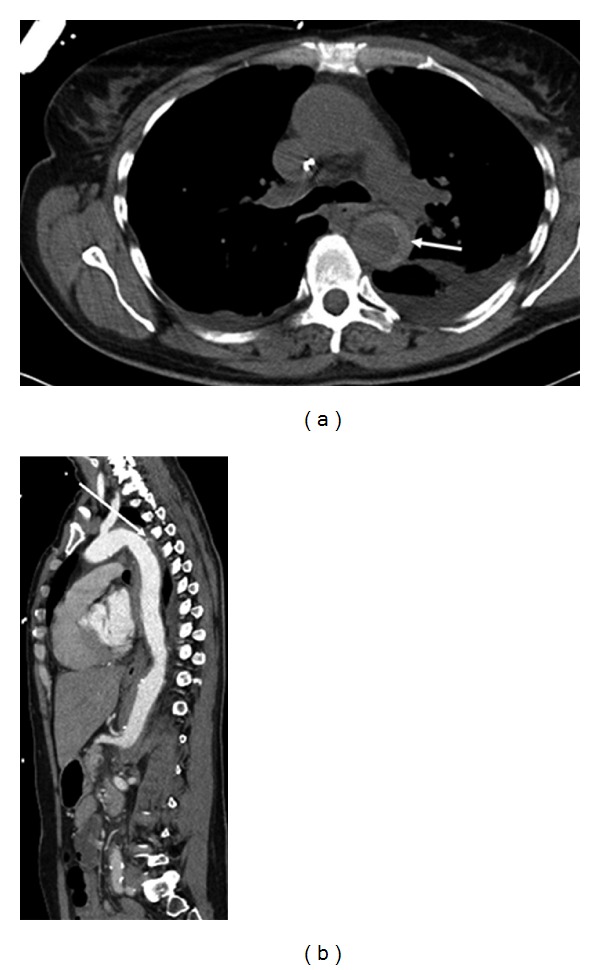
(a) Nonenhanced CT depicting intramural hematoma (arrow). (b) Sagittal reconstruction of CT-angiography (CTA) with penetrating ulcer (arrow).
